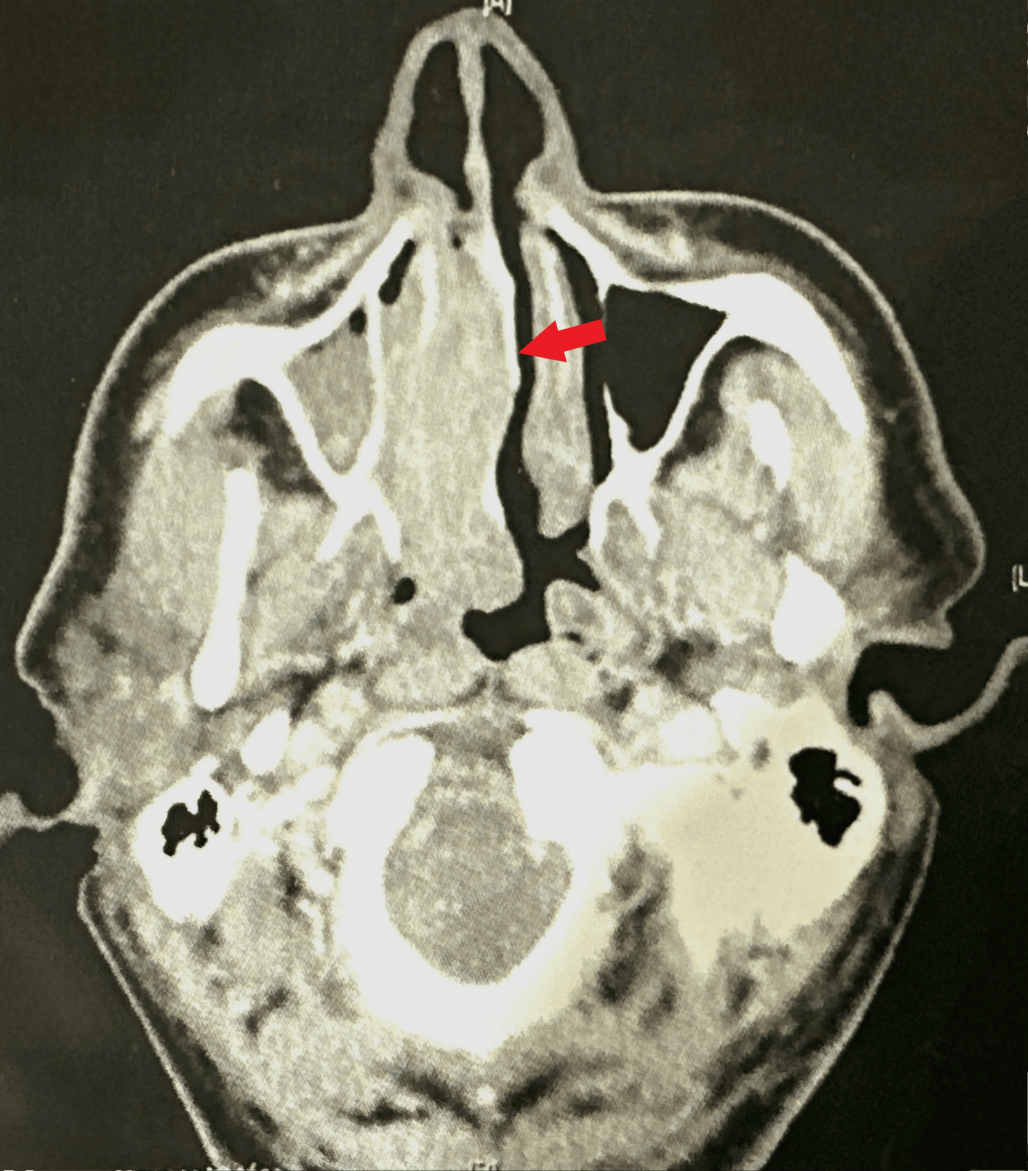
CT scan of the facial mass in the parenchymal window axial section without contrast injection: tumor process in the right antro-choanal fossa measuring (6 x 5 x 2.7 cm), with bone lysis of the nasal septum.
